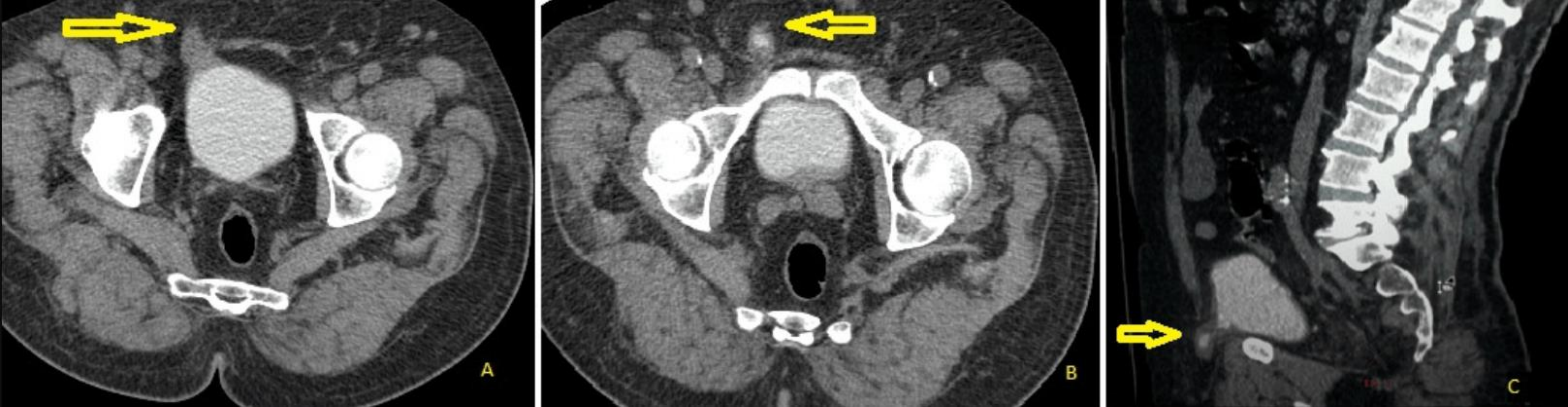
A-C) CT scan showing right direct inguinal hernia containing a bladder horn in axial and sagittal views
